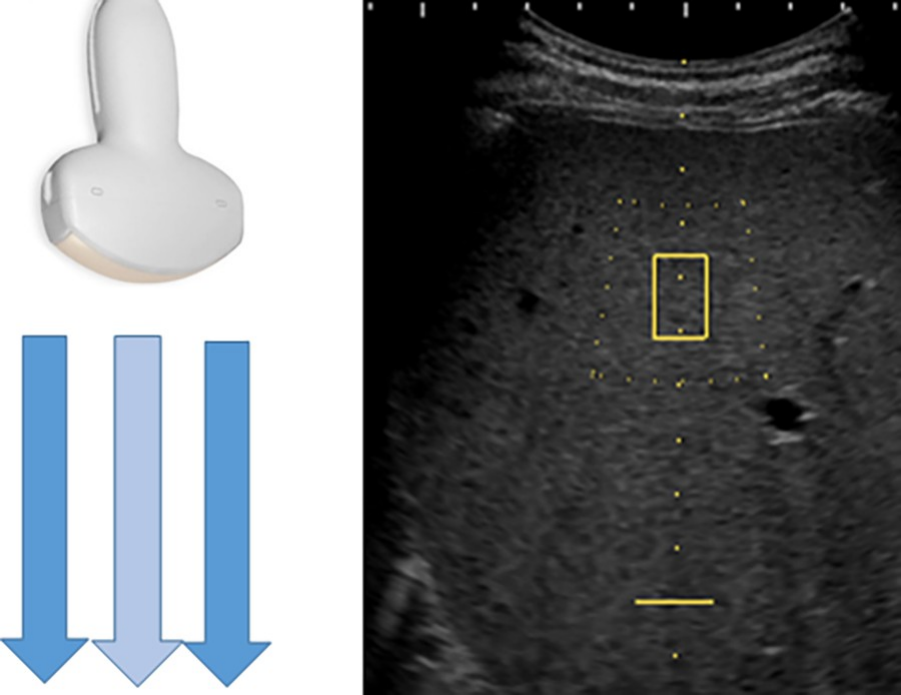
Diagnosis of fatty liver using attenuation in the B mode. Multiple ultrasound waves with different frequency components are used when measuring in the B mode. Fat volume estimation is performed according to the difference in the degree of attenuation of the received signal. Measurement is performed while observing the relevant part in real time.
